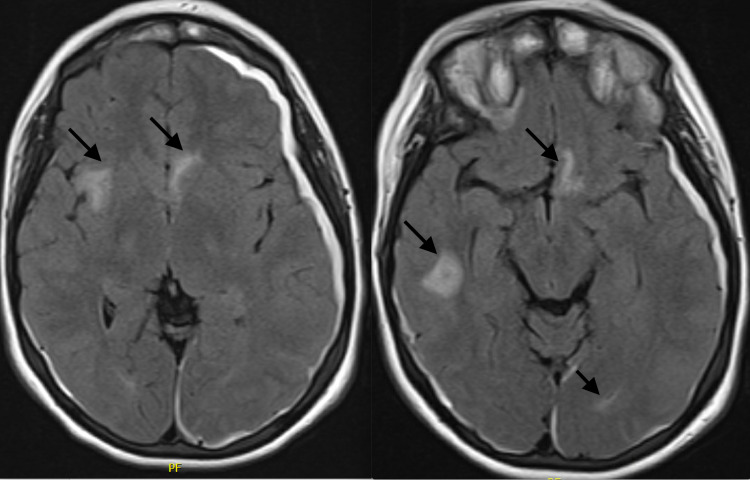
MRI findings: Similar findings to CT scan. Arrows in both images pointing to multifocal areas of intracranial hemorrhage
